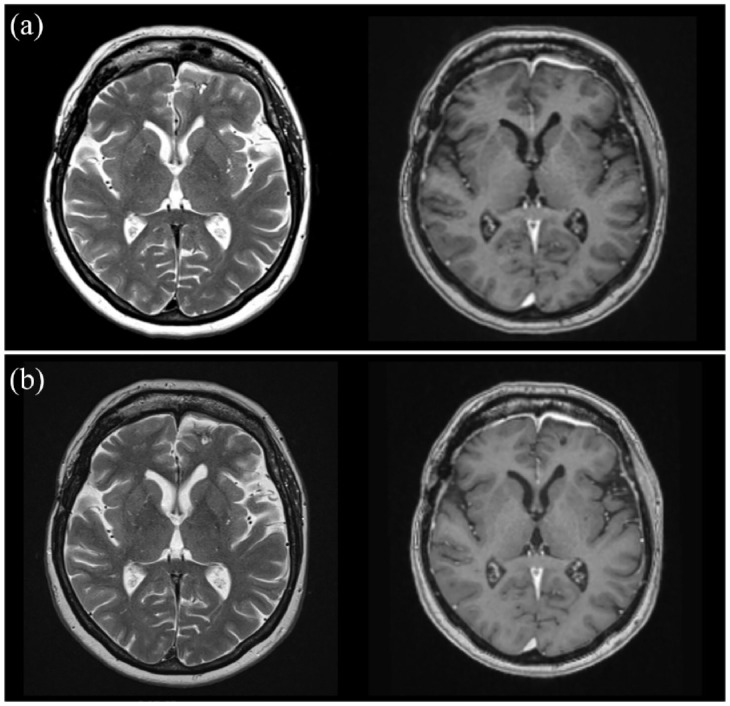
Magnetic resonance imaging findings (a) 3 months and (b) 7 months after initiation of the treatment.
